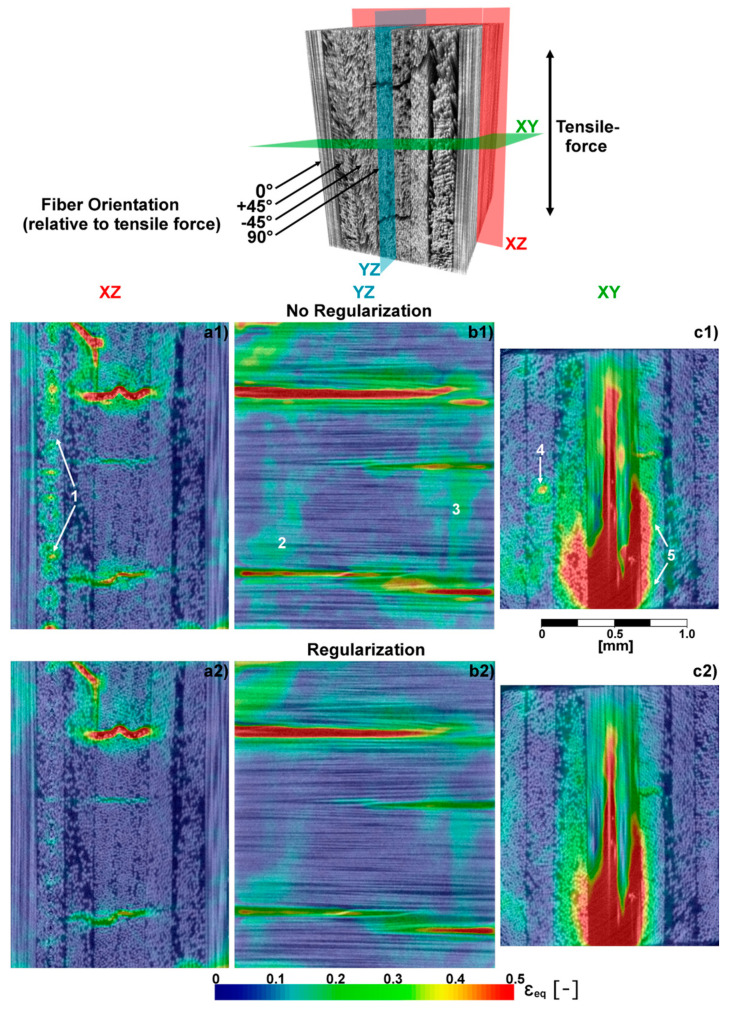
Top: Volume rendering with fiber orientation and force indicator. The coordinate system is adopted from Figure 4. Colored planes represent the positions of the 2D images shown in (a1–c2); (a1–c2) contain von-Mises equivalent strain quantities superimposed on the respective CT-slices. Landmarks (1–5) in (a1–c1) emphasize locations of ambivalent and erroneous strain quantities distinctly reduced by the implementation of the regularization (see (a2–c2)).
